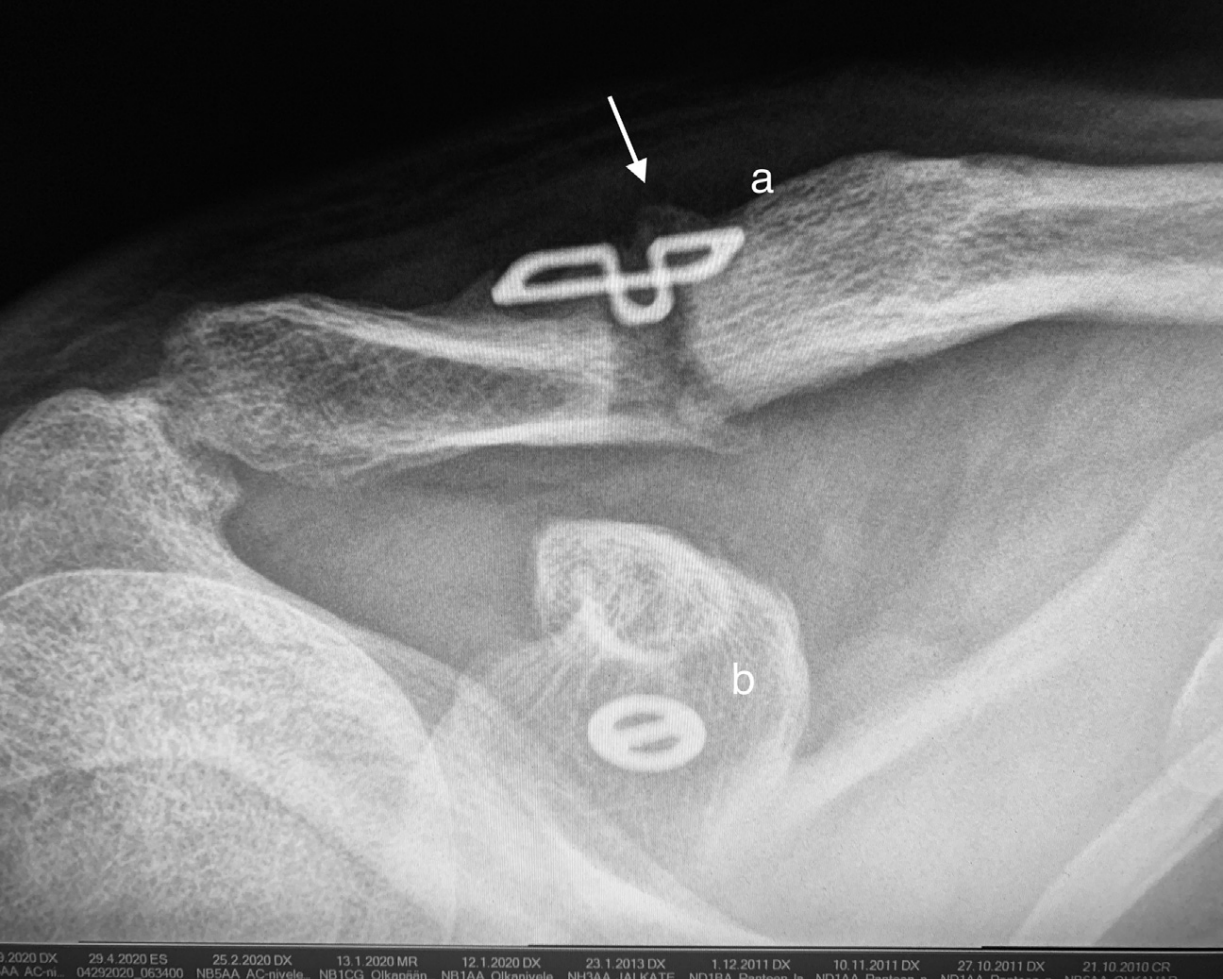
A clavicular fracture occurred in ice-hockey 8 months after the operation. The fracture line went through the clavicular drill hole. The fracture was treated conservatively. The fracture healed, and the tendon reconstruction remained in place. The Clavicular Clip (a), the Subcoracoid Clip (b) is shown. Regenerative bone on the clavicle (arrow). An anteroposterior radiograph of the healed right shoulder 4 months after injury.
